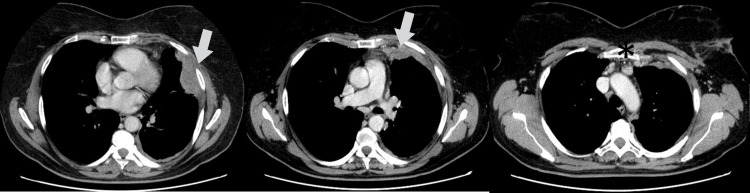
Computed tomography scan (May 2021) - pleural thickness (arrows) and 15 mm adenomegaly (*)
